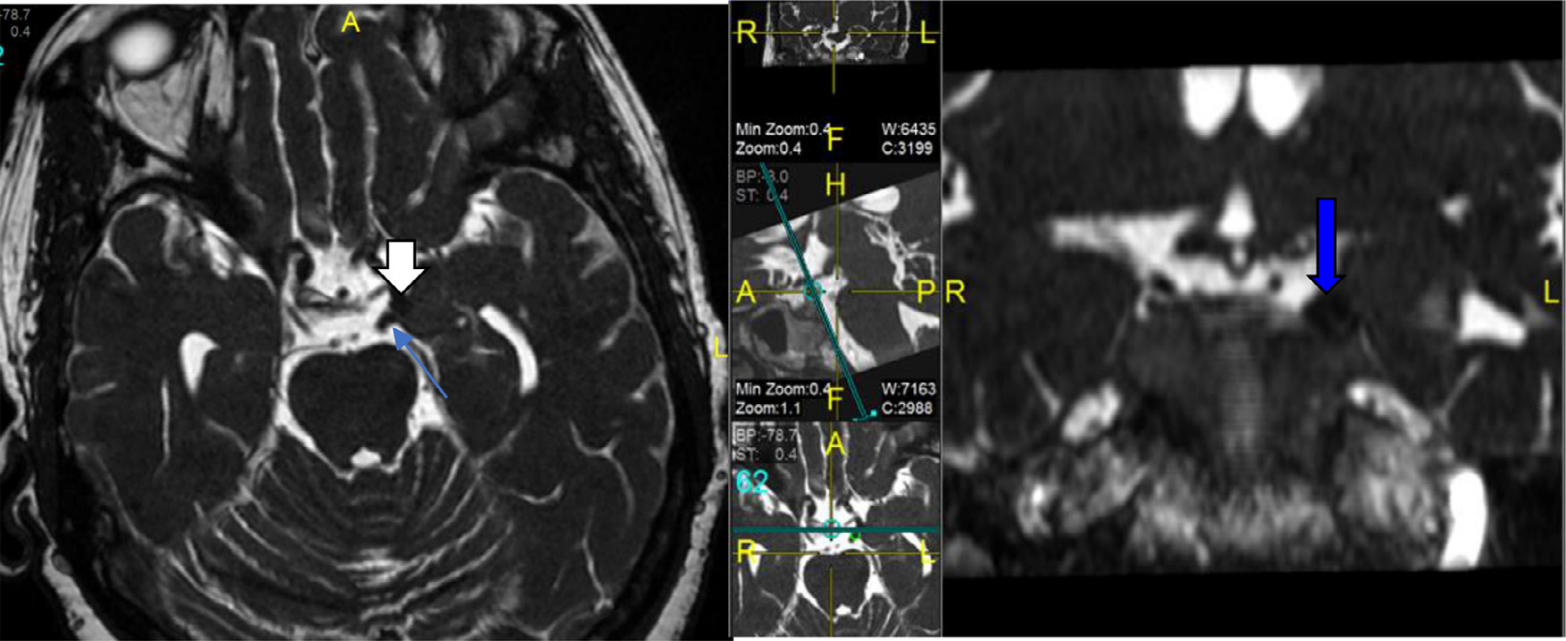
Axial and coronal FIESTA sequence magnetic resonance image demonstrating compression of left oculomotor nerve (blue arrow) by saccular aneurysm (white arrow) (color version of figure is available online.)
